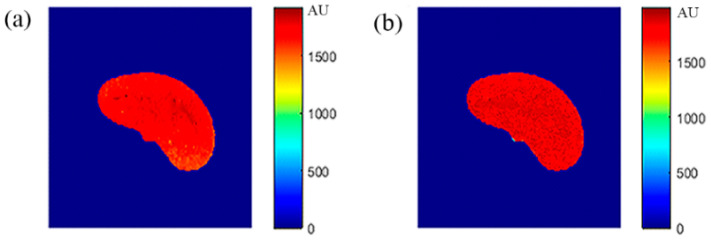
(a) The real MR image with *T_E_* = 10 ms and *T_R_* = 4000 ms, and (b) the generated synthetic MR image using the same *T_E_* and *T_R_* values.
